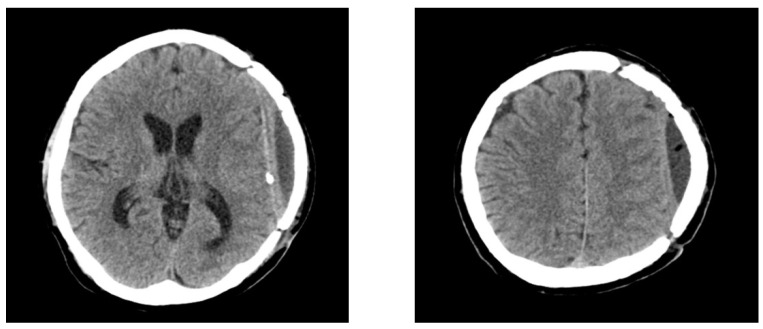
Brain computed tomography scans at 8 months after cranioplasty reveal improvement in the air bubbles and epidural fluid collection. However, the patient did not require any additional procedure due to the absence of neurological abnormalities, and a follow-up CT scan performed 8 months after the cranioplasty demonstrated decreased epidural fluid collection without midline shift (Figure 2).
